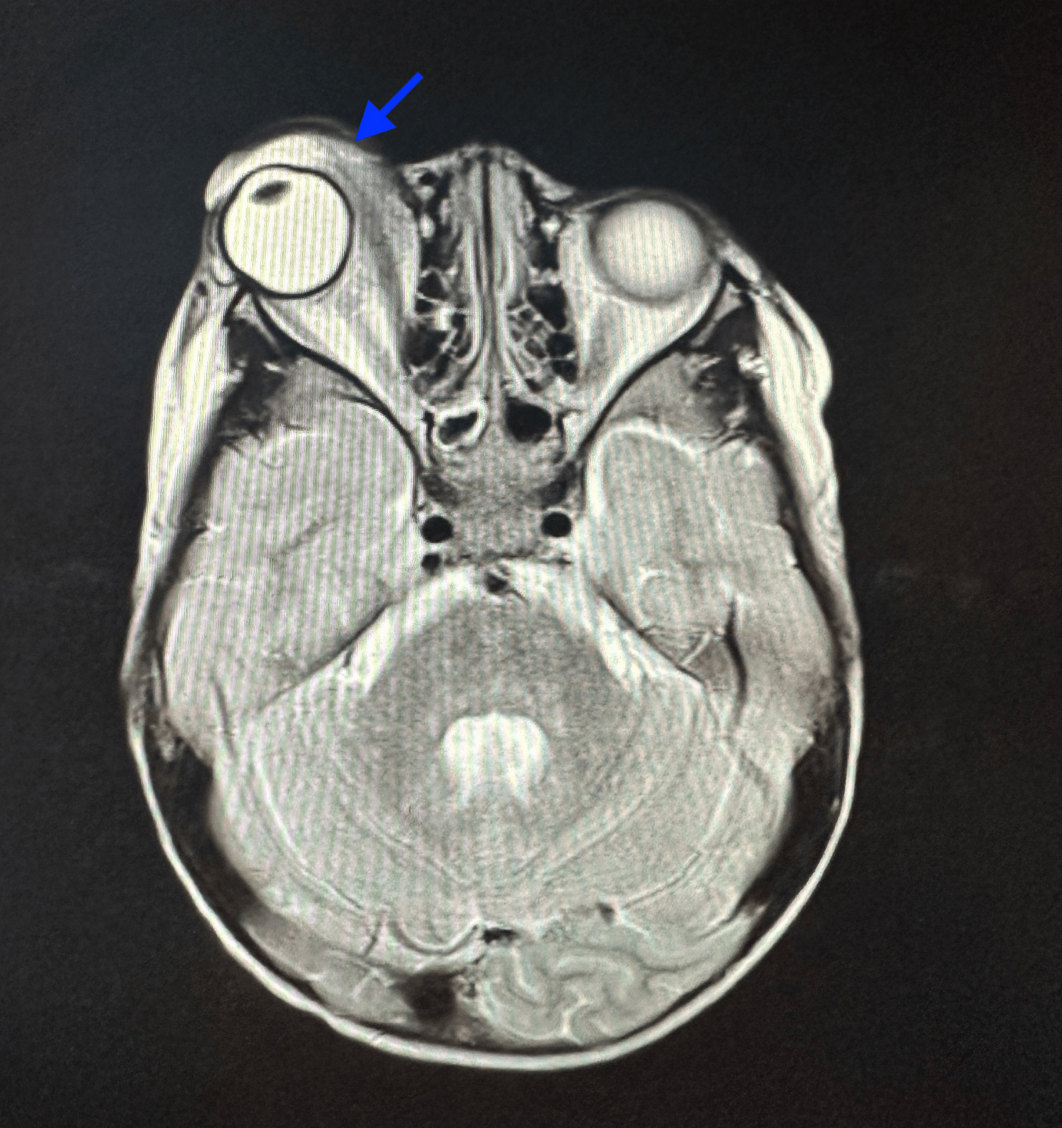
Axial T2-weighted MRI scan showing a heterogenous lobulated solid mass at superomedial aspect of the right orbit, displacing the globe inferolaterally (blue arrow) MRI: magnetic resonance imaging
